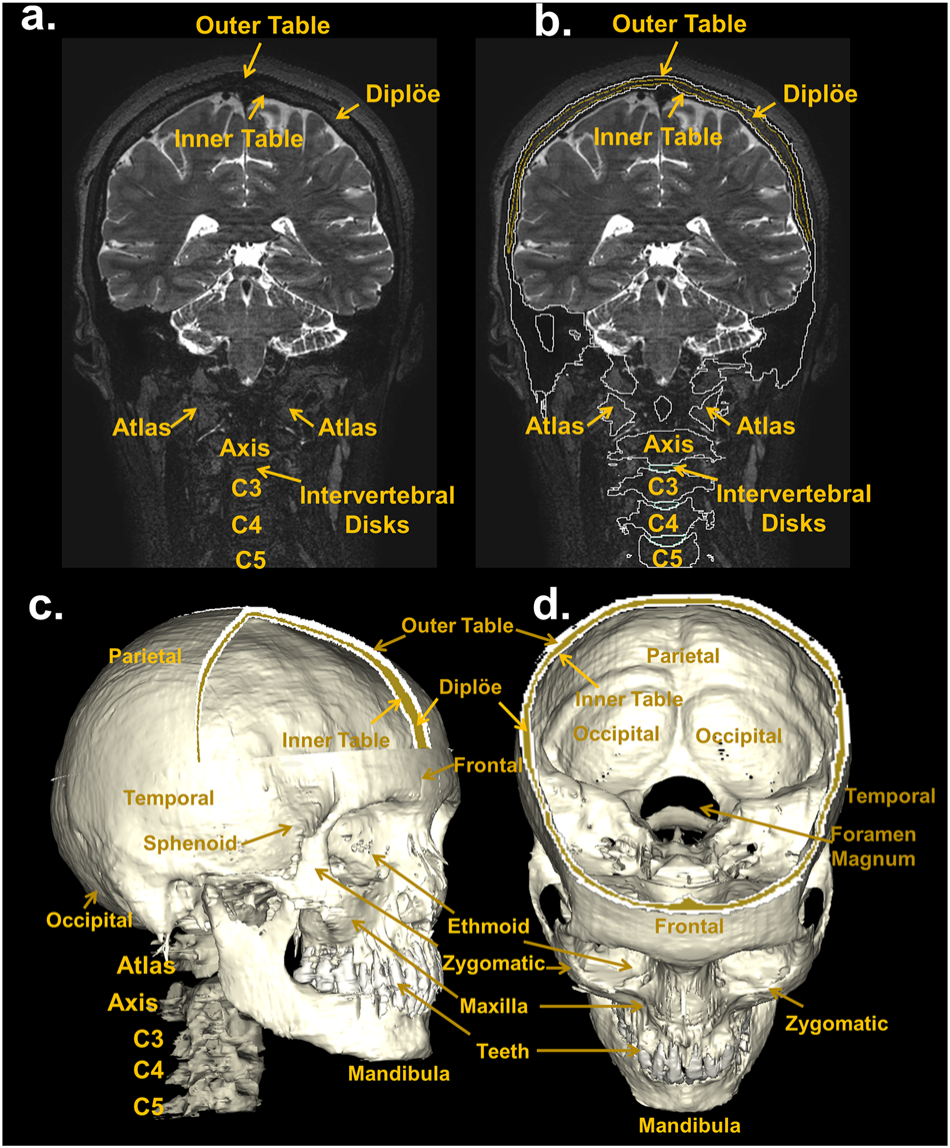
Segmentation of the bone. Top: coronal view of a T2-weighted MRI slice (a) with the skull, vertebrae, and intervertebral disks outlined (b). The T2-weighted MRI, in which the intensity of the cancellous bone inside the diploë is enhanced compared to that of the cortical bones of the inner and outer tables made the subdivision of the skull into the main three layers, i.e., outer table (white outline), diploë (yellow outline), and inner table (white outline) possible. Bottom: 3D reconstruction of the skull, vertebrae, and intervertebral disks (c and d).
